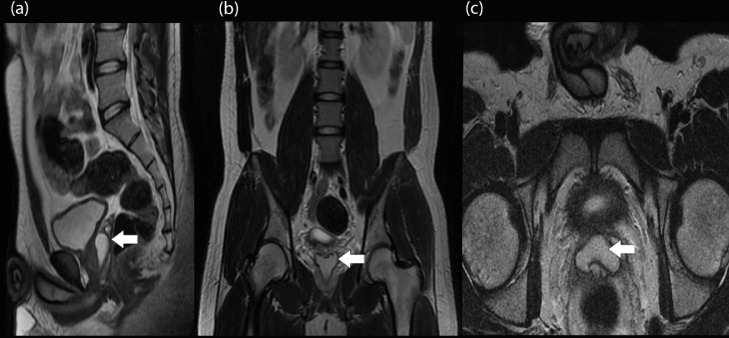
Contrast-Enhanced abdominal MRI showing a hyperintense lesion on TW1, indicating a cyst from the (A) sagittal, (B) coronal, and (C) axial views, as shown by the white arrows.
